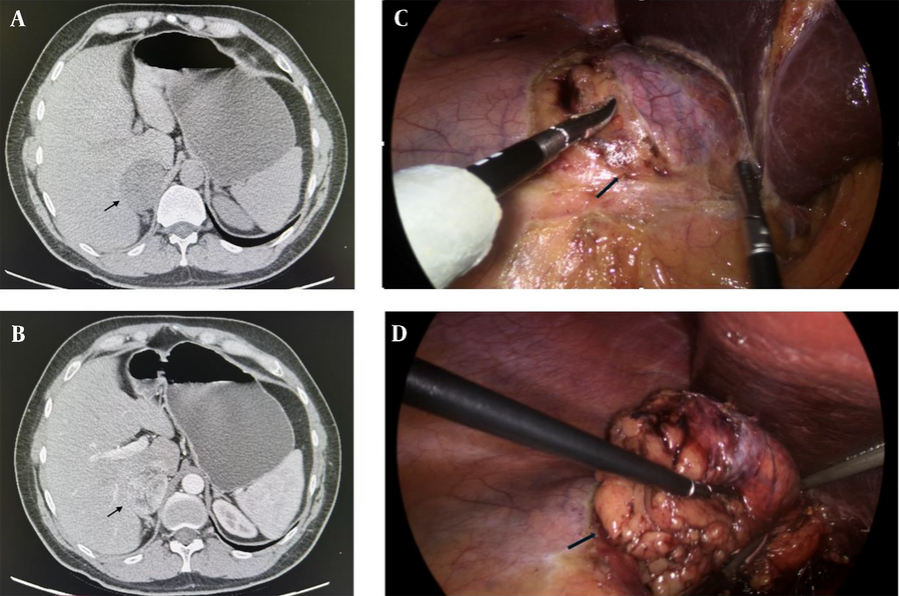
A and B, multi-slice computed tomography (MSCT) scan of the abdomen, transversal section, arrows show a right adrenal gland tumor measuring 57 × 47 × 59 mm (arrow). A, both native; and B, arterial phases are shown; C and D, intraoperative finding; C, dissection phase- exposition of the right adrenal gland tumor (arrow); D, end of dissection and extraction of the tumor a right adrenal gland tumor measuring 5 cm (arrow).
